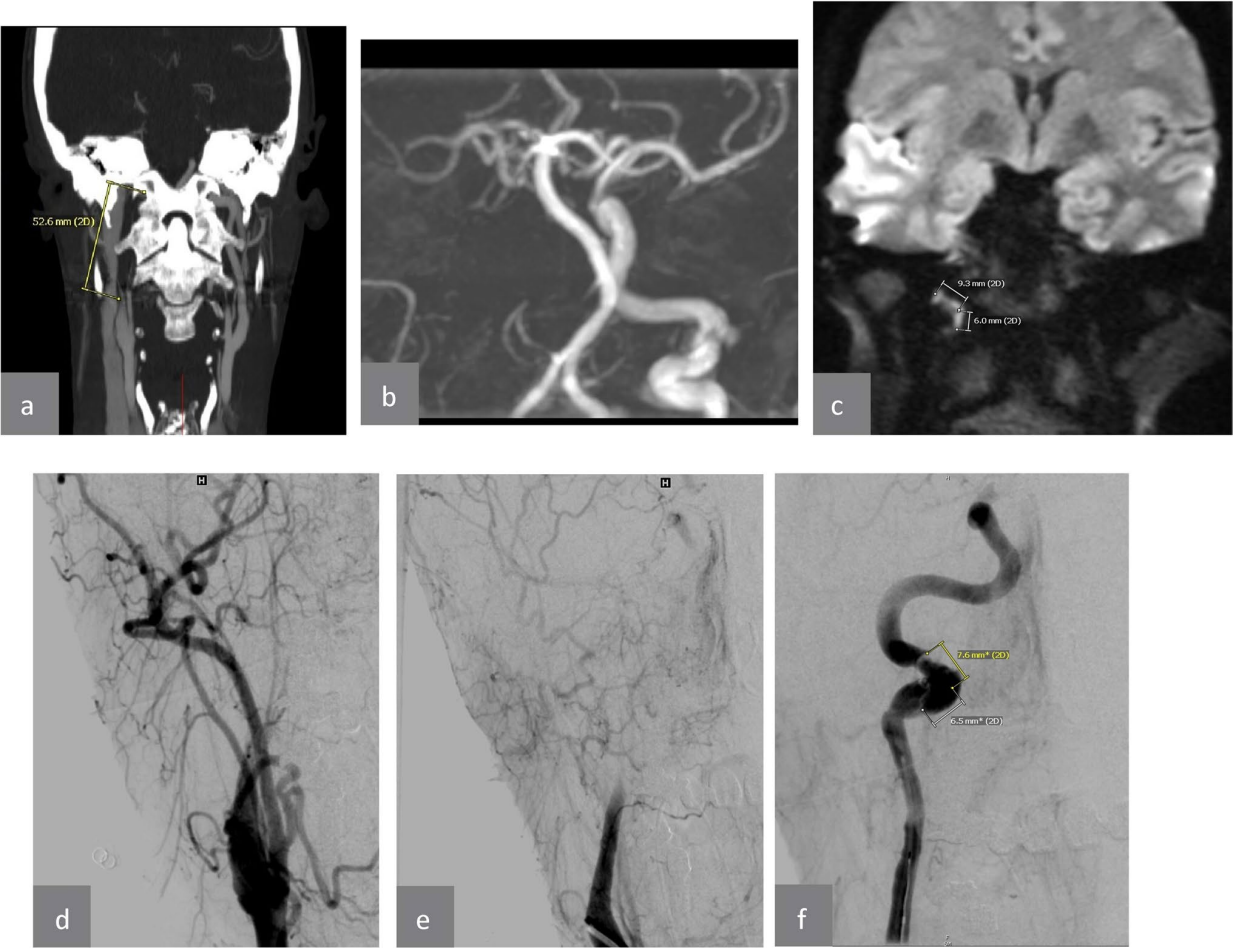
A case with DSA, CTA, MRA and DWI that shows that CTA and MRA were misleading and that DWI matched DSA. a A coronal CTA shows a contrast agent stop immediately distal the ICA origin that supposes a long distance occlusion. b A coronal TOF reconstruction shows a long distance absent flow signal in the right ICA, which supposes also a long distance occlusion. c A coronal DWI in contrary to the previous modalities shows a short distance hyperintensity in the ICA wall at the skull base. d A coronal DSA shows a contrast agent stop immediately distal the ICA origin, while the catheter tip was still located in the common carotid artery (CCA). e A coronal DSA shows contrast agent more distal and in a longer segment of the ICA after the catheter tip was placed in the ICA origin. f A coronal DSA after replacing the catheter and moving it carefully until there was a resistance against forward catheter pushing that shows now the real dissected segment of the ICA, which matches the length of DWI hyperintensity
